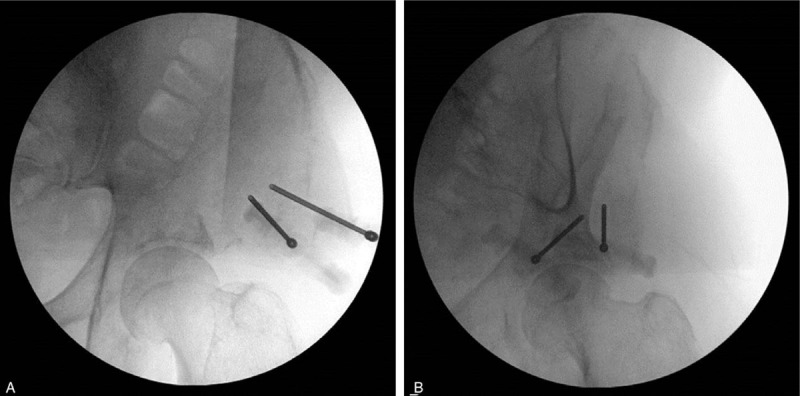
Left pelvic radiograph obtained using C-arm fluoroscopy in the operating room. Anteroposterior view (A) and oblique view (B) show the placement of the needle in the osteolytic lesion.
